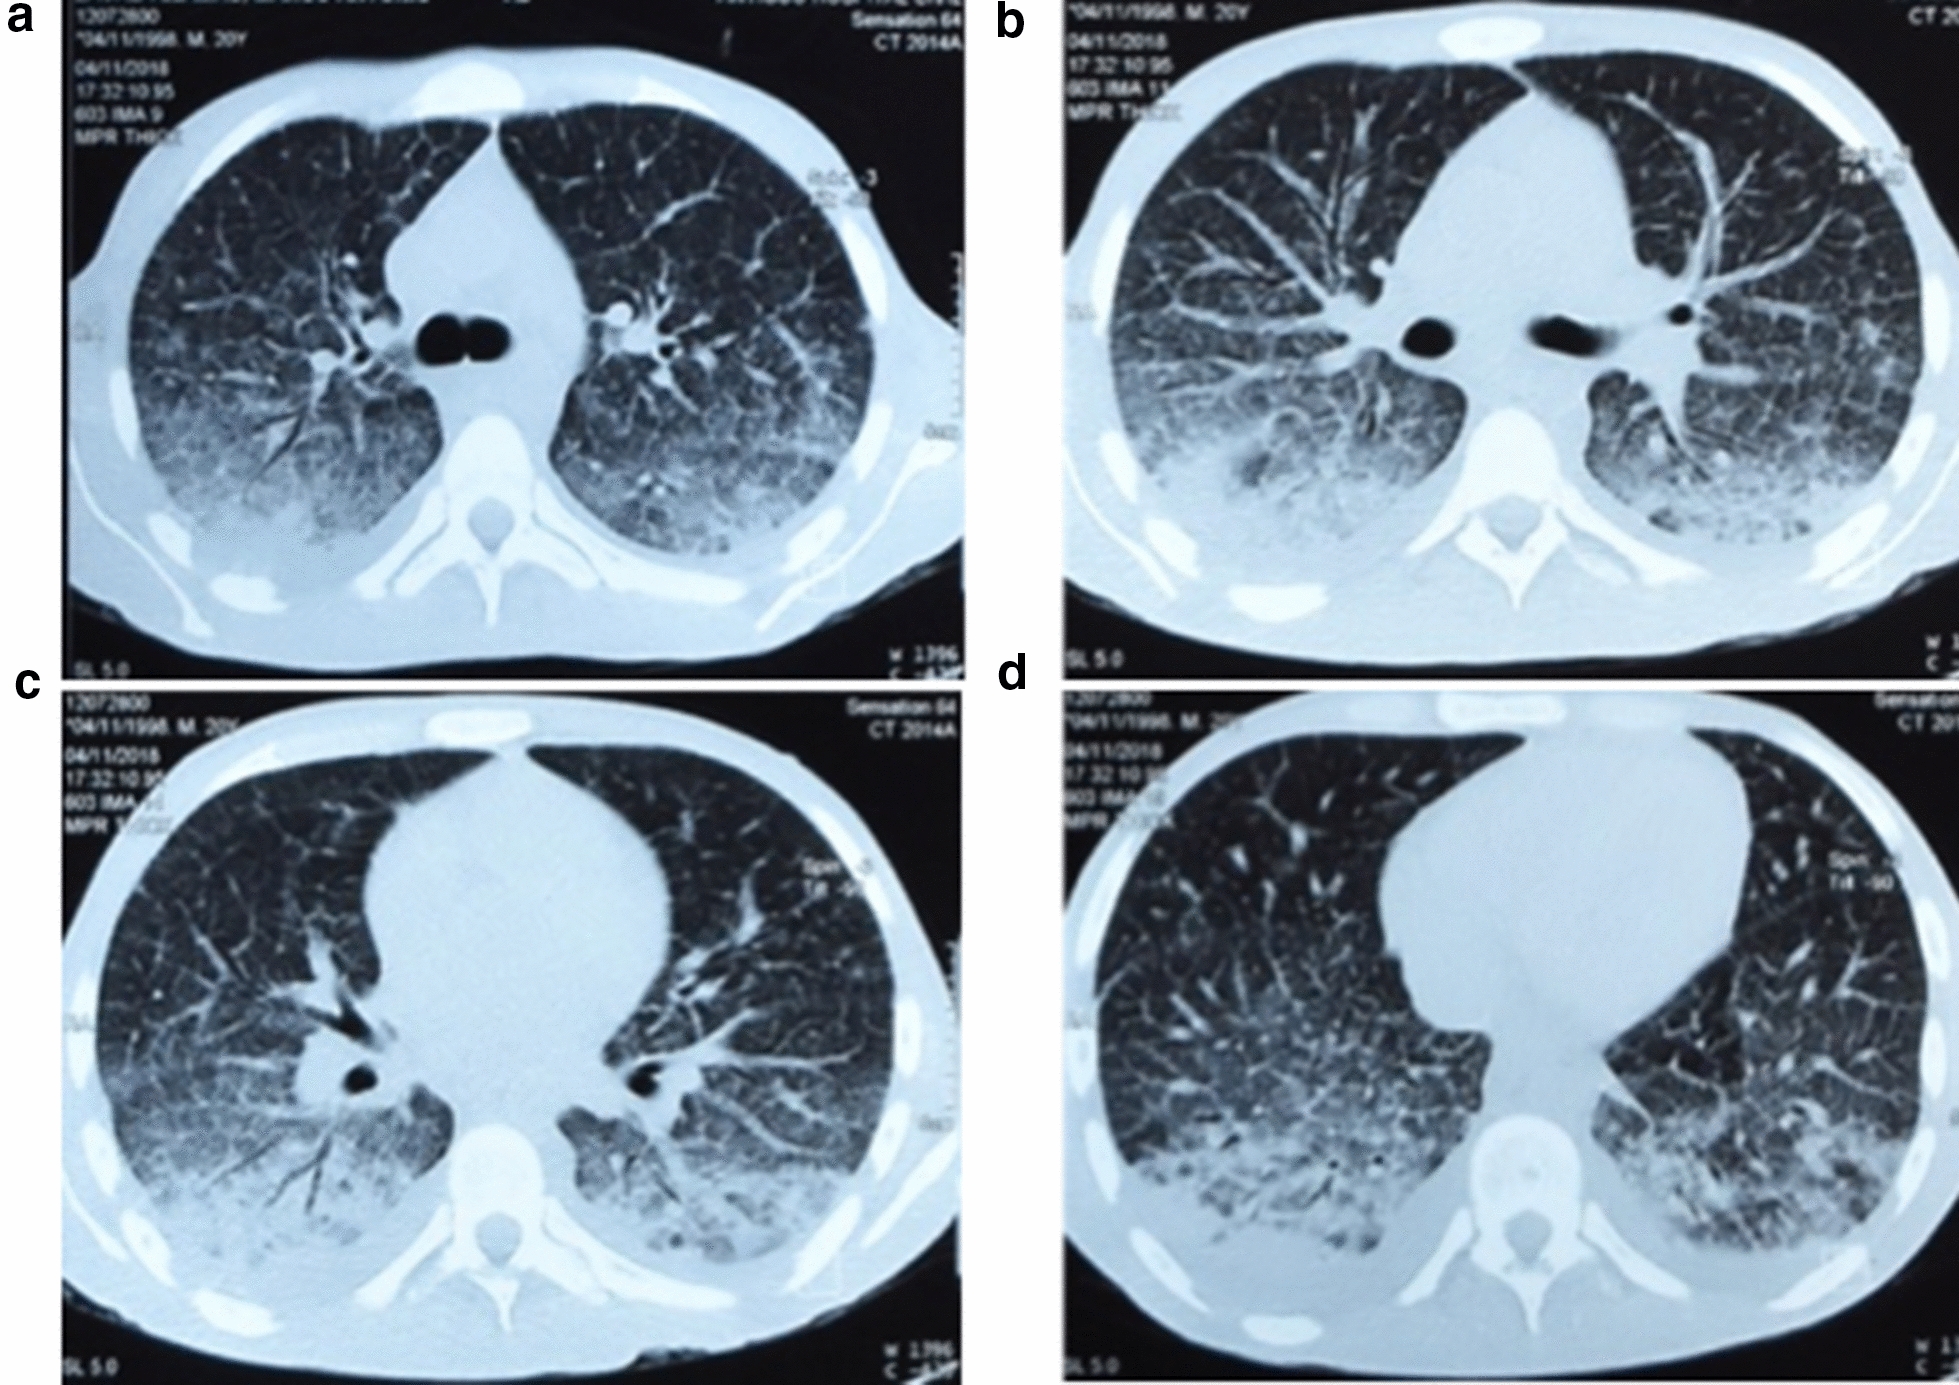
a–d A chest computed tomography shows bilateral basal consolidation areas, interstitial and reticulonodular infiltrates at different levels
